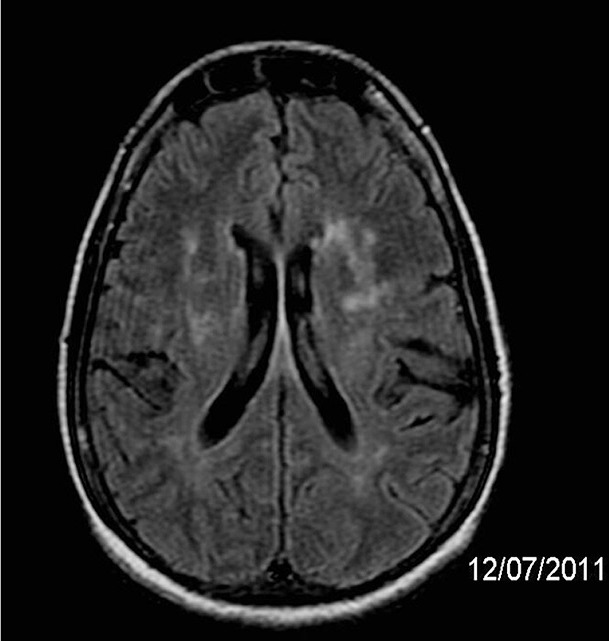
Brain Nuclear Magnetic Resonance Images of the axial planes using FLAIR
